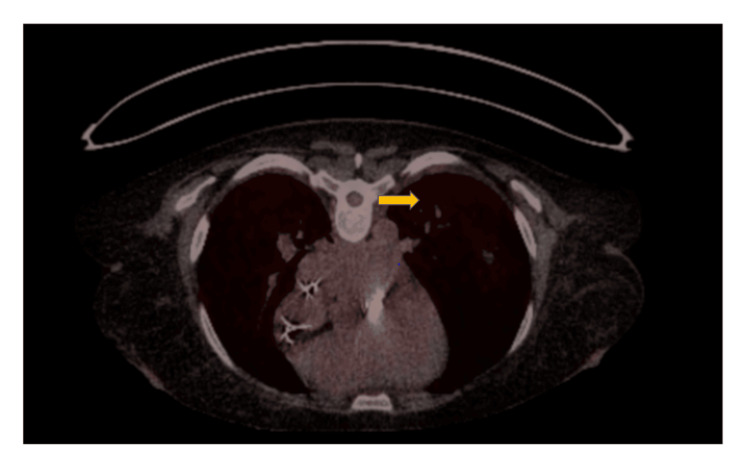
Positron emission tomography/computed tomography (PET/CT) of lungs Arrow indicates pneumonia in left lung
